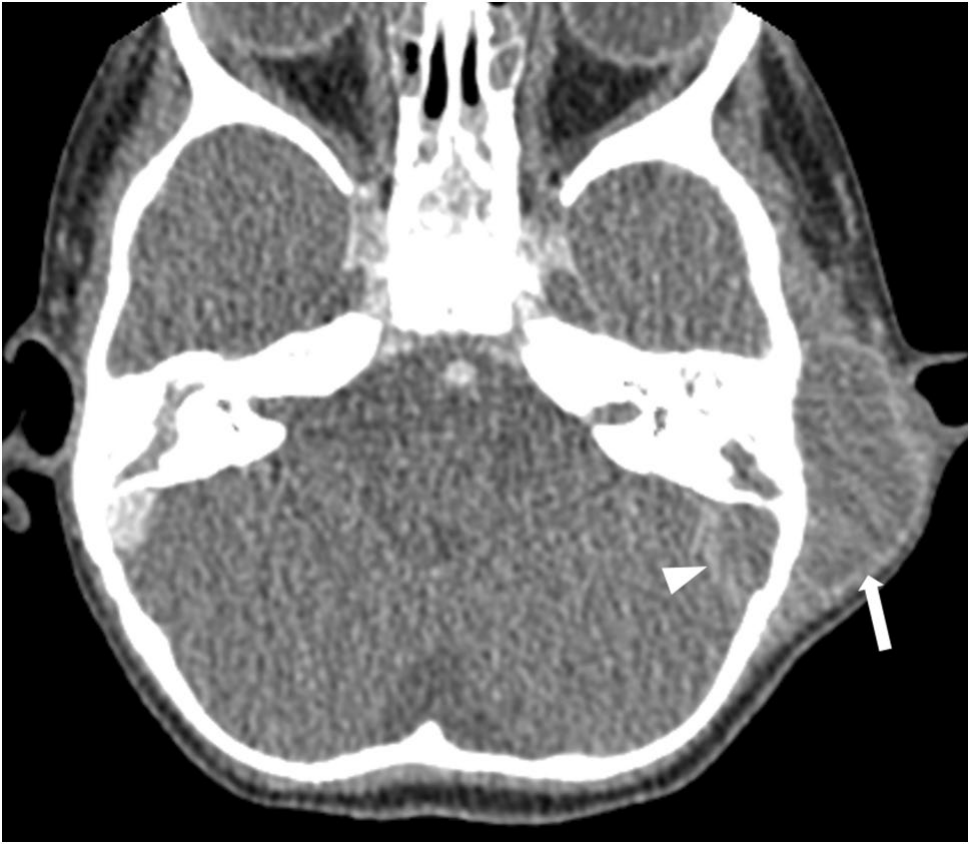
Axial post-contrast computed tomography image (soft tissue window) in a 2-year-old girl with a sinus vein thrombosis in the left sigmoid sinus (*arrowhead*) and a retroauricular abscess (*arrow*) caused by mastoiditis
